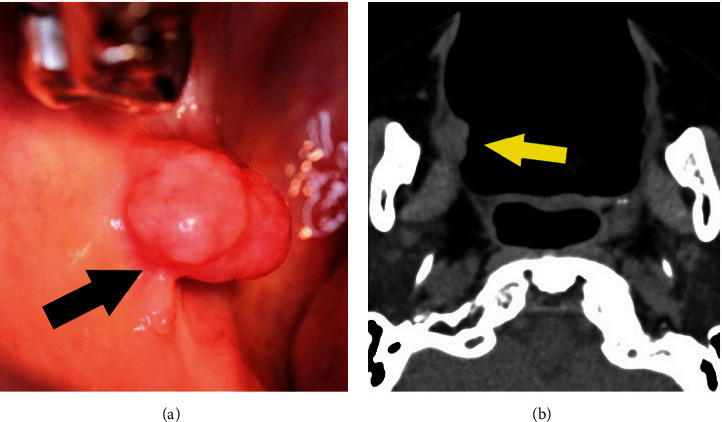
Intraoral appearance of the buccal mucosa and CT image. (a) The mucosal epithelium was normal in color with a smooth surface. There was no induration around the tumor (black arrow). (b) CT (axial view) showed an enhanced lesion on the anterior portion of the right palatoglossal arch (yellow arrow).
